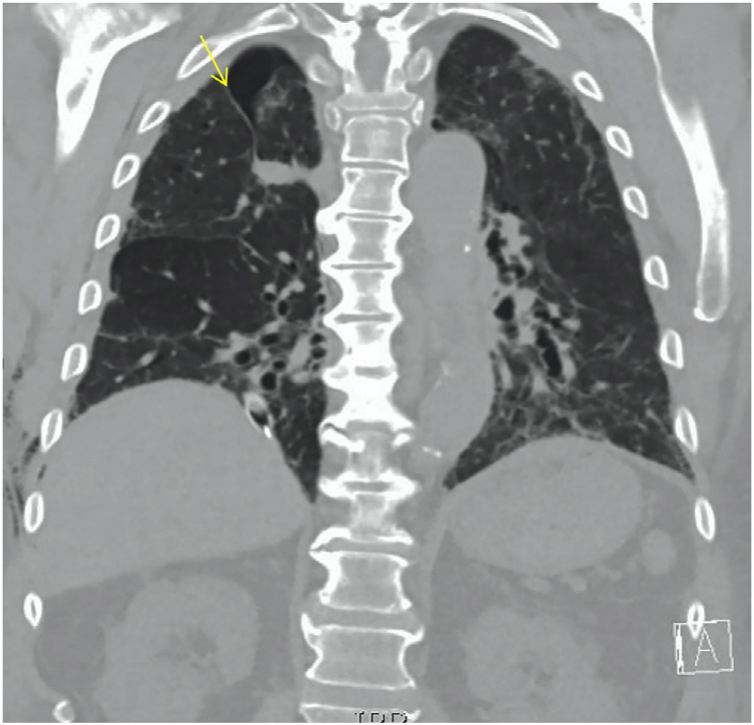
Chest computed tomography revealing several bullae and azygos fissure (arrow).
